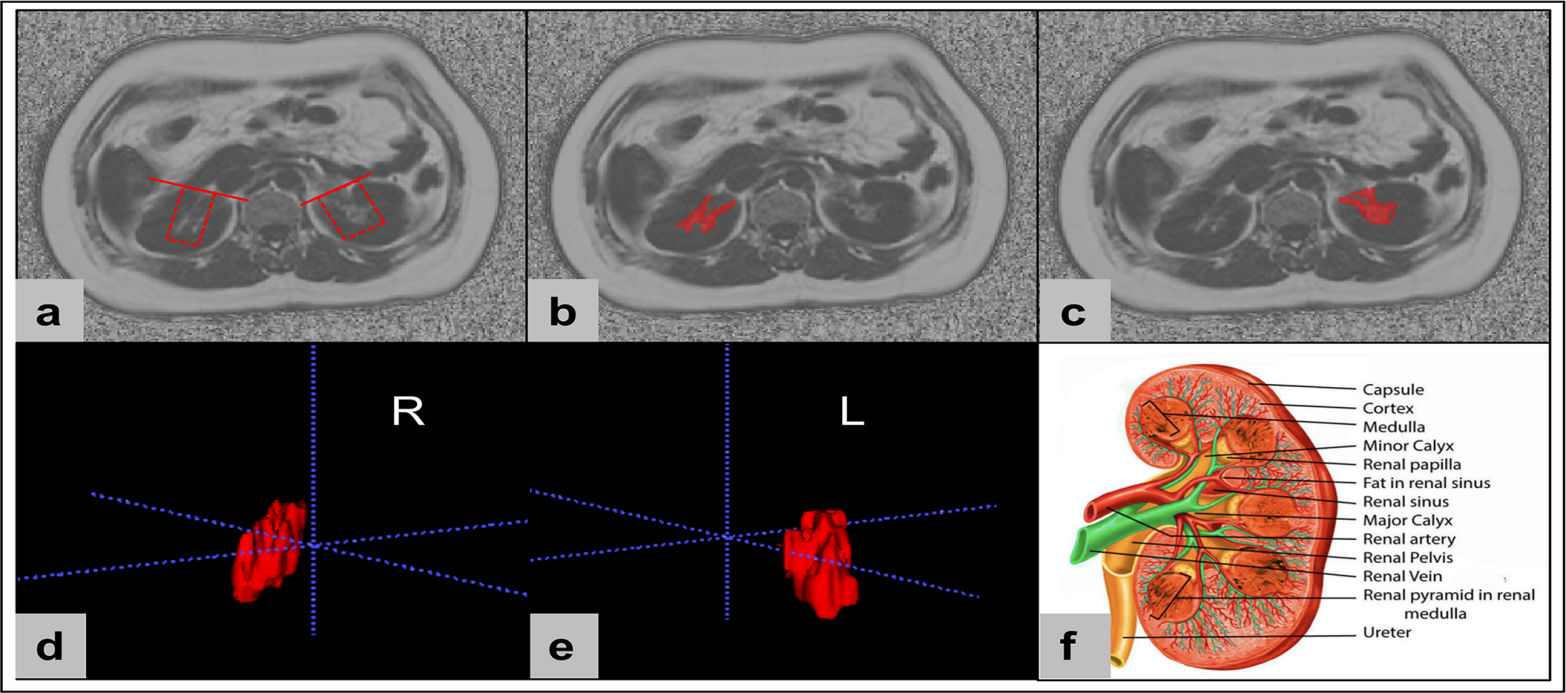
The renal sinus fat was manually conducted using the open-source software ITK-SNAP (v.3.6.0, http://www.itksnap.org/). Renal sinus fat of both kidneys was identified on MRI fat fraction maps by a straight-line tangent to the margins of parenchyma beside the renal hilum in axial slices (A). Then the bilateral renal sinus adipose tissues were manually segment with structures of renal lymphatics, veins, and the ureters removed (B, C). Finally, the renal sinus fat volume and FF of right and left kidneys were automatically calculated based on the 3D segmentations (D, E). Sketch of the renal anatomy (F) (Source: [21] distributed under the terms of the Creative Commons Attribution 4.0 International License (https://reativecommons.org/licenses/by/4.0/, accessed on the 1 March 2023) © 2023, StatPearls Publishing LLC).
